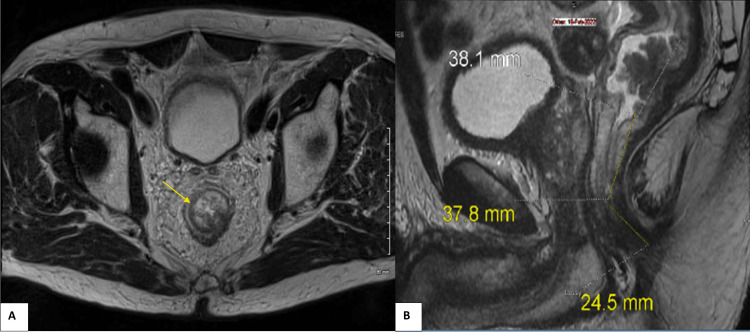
MRI of the pelvis without contrast undertaken for local rectal cancer staging The following T2-weighted MRI images demonstrate a polypoid mass (A) (arrow) in the mid-rectum with a craniocaudal length of about 3.8 cm (B). MRI: magnetic resonance imaging
